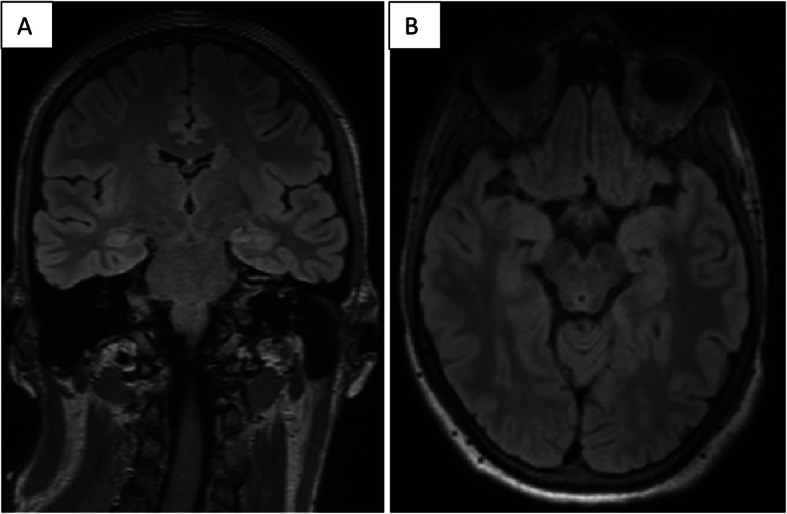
Coronal (A) and axial (B) FLAIR MRI sequences. No lesions could be disclosed during the acute phase nor during follow-up
